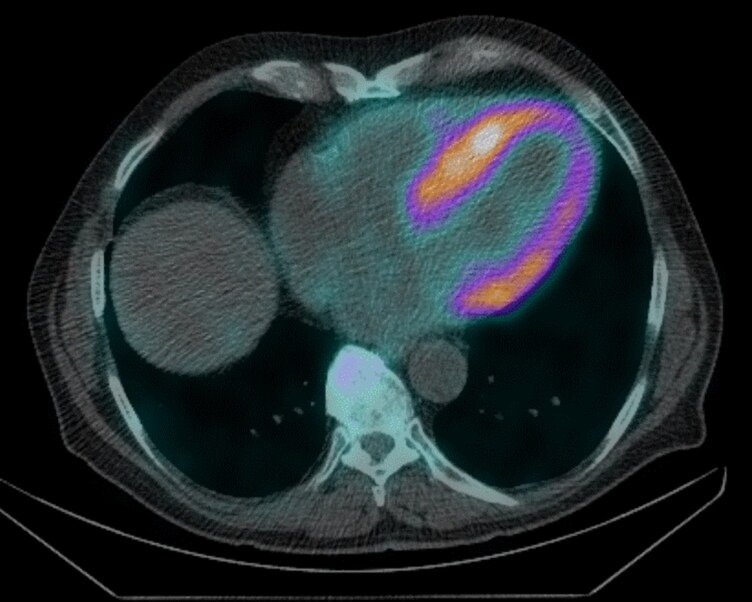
DPD scintigraphy with single-photon emission computed tomography (SPECT/CT) imaging demonstrating tracer accumulation predominantly in the basal LV wall.
